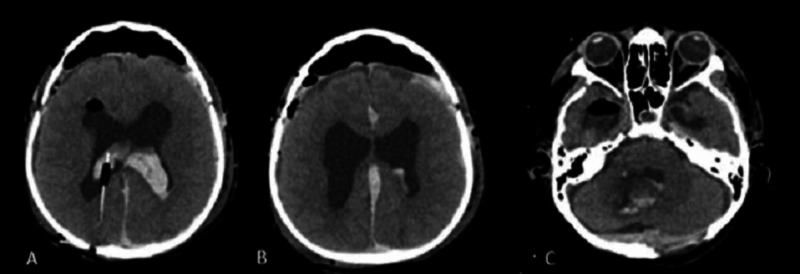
Non-contrast Brain CT scan at day 4, axial view A: Intraventricular hemorrhage. B: interhemispheric bleeding. C: Hemorrhage in the fourth ventricle.
